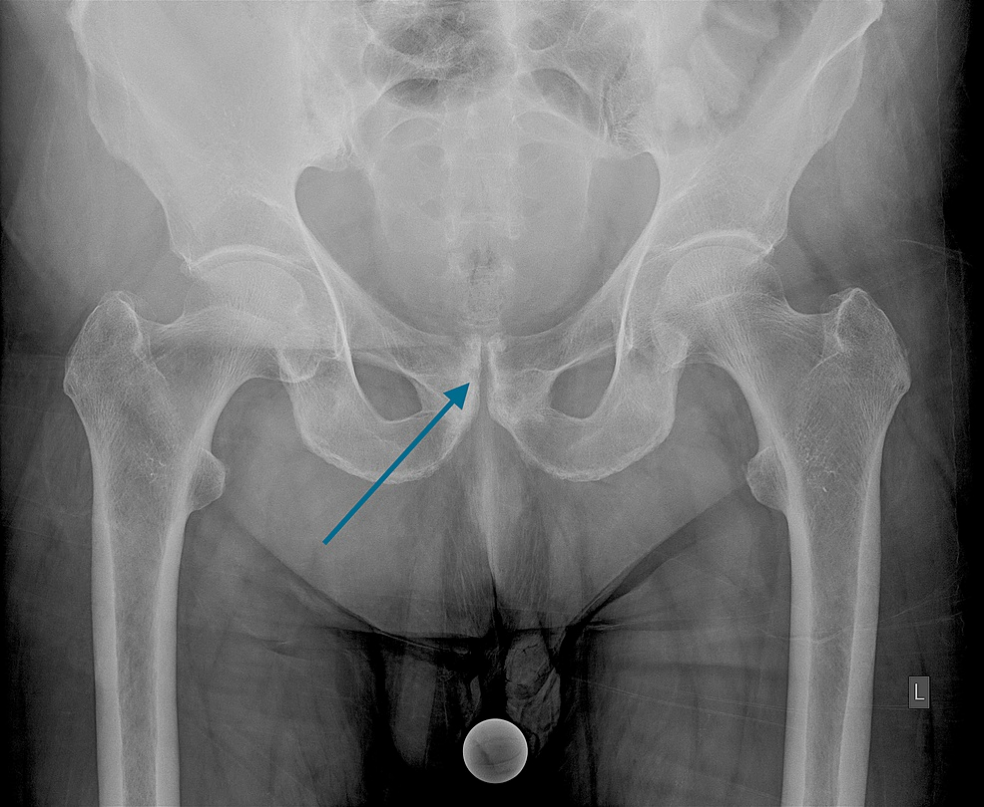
Anteroposterior radiograph of the pelvis at presentation with subtle erosive changes of the symphysis (arrow).
